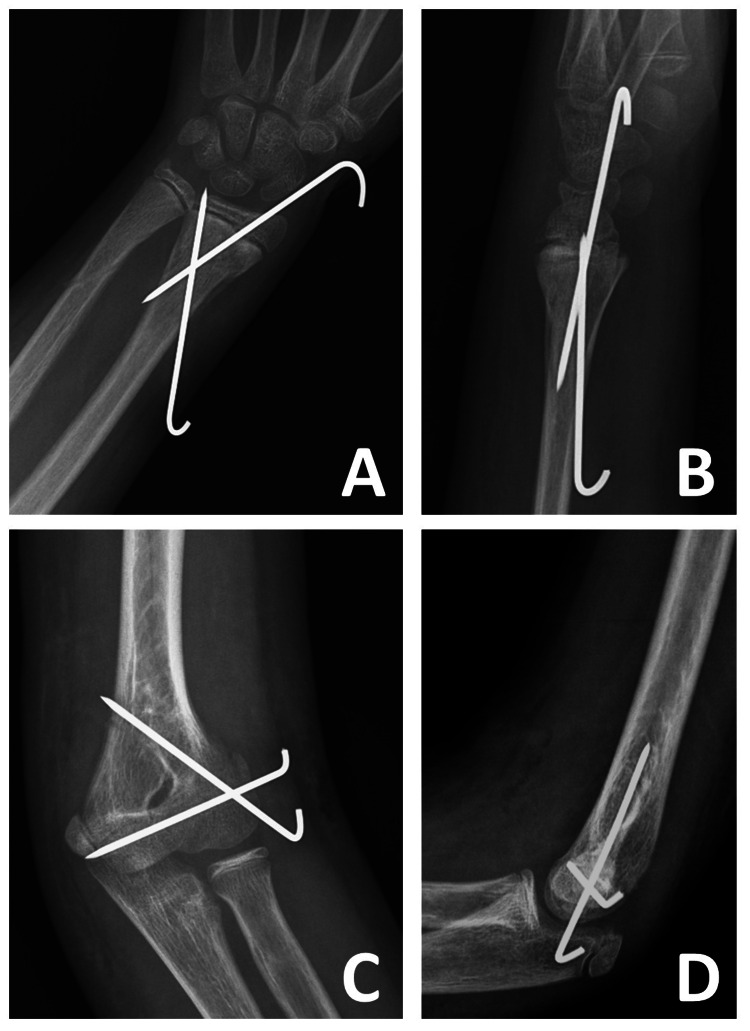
Radiographs taken at four weeks postoperatively (A, B) Anteroposterior and lateral radiographs of the left wrist. (C) Internal oblique radiograph of the left elbow. (D) Lateral radiograph of the left elbow.
